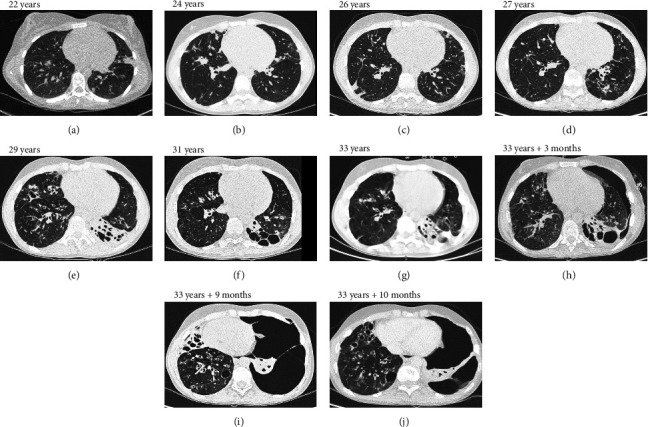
chest computed tomography scan at age 22 years, as well as multiple scans during follow up over the years. The scan at age 33 years was made when the patient presented to the emergency department with COVID-19. (a) Bilateral consolidations that are not well circumscribed, multiple nodules, fissural and pleural irregularities and multiple ground glass areas. Diffuse thickening of bronchial walls. Overall appearance suggestive of granulomatous disease. (b) Disappearance of multiple consolidations, and appearance of multiple new consolidations and ground glass abnormalities. (c) Disappearance of some consolidations in the lower fields, but appearance of new consolidations in the upper fields. (d) Disappearance of some consolidations and appearance of new consolidations and ground glass. (e) New consolidation in the left lower lobe. (f) Impressive progression of bronchiectasis in the left lower lobe, disappearance and appearance of consolidation and ground glass. (g) New consolidations and ground glass, mainly in the lower fields. (h) Pneumothorax on the left side, increase in ground glass and consolidations in both lungs. (i) Increase in pneumothorax on the left side, mediastinal shift to the right, increased bronchiectasis in the middle lobe. (j) Increased air in left upper lobe.
